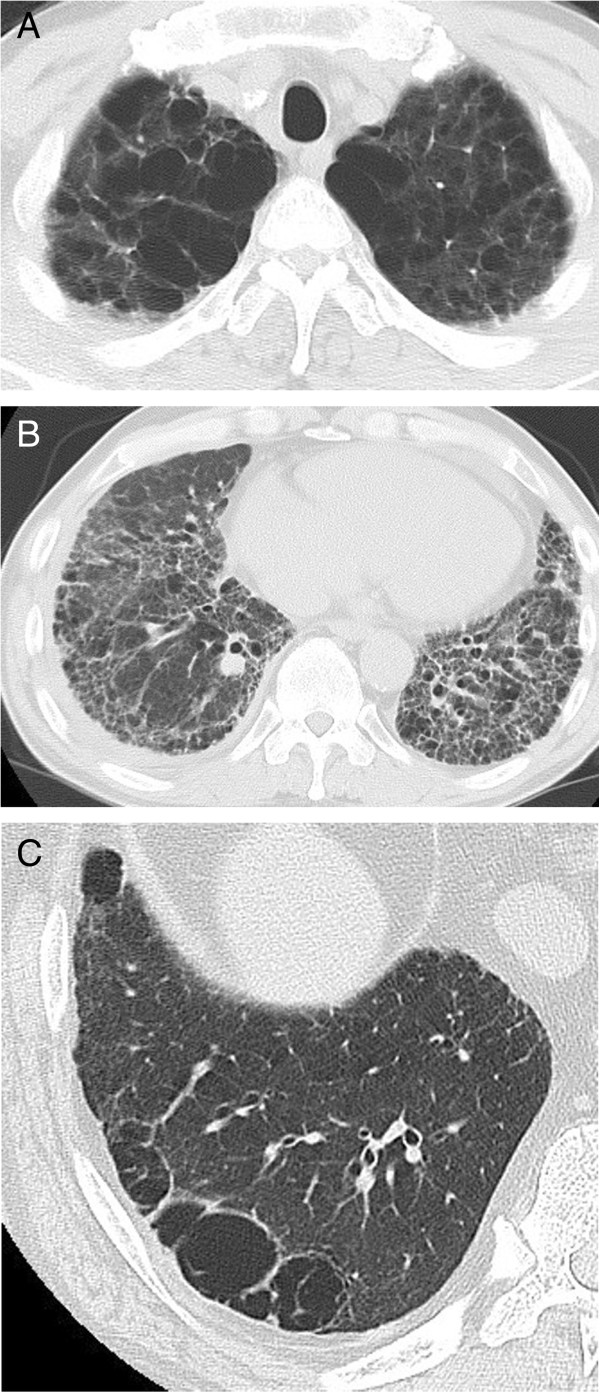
Representative computed tomography (CT) scans performed within 1 month of death in a patient with combined pulmonary fibrosis and emphysema (CPFE) without lung cancer who died from pulmonary hypertension (A, B), and in another such patient with lung cancer who died from a non-respiratory cause (C). (A) Upper lobe showing centrilobular and paraseptal emphysema and bullae. (B) Lower lobe showing reticular opacities with peripheral and basal predominance and honeycombing, which was diagnosed as definite usual interstitial pneumonia (UIP) pattern. (C) Lower lobe showing thick-walled cystic lesions (TWCLs) larger than honeycombing with peripheral reticular opacities.
